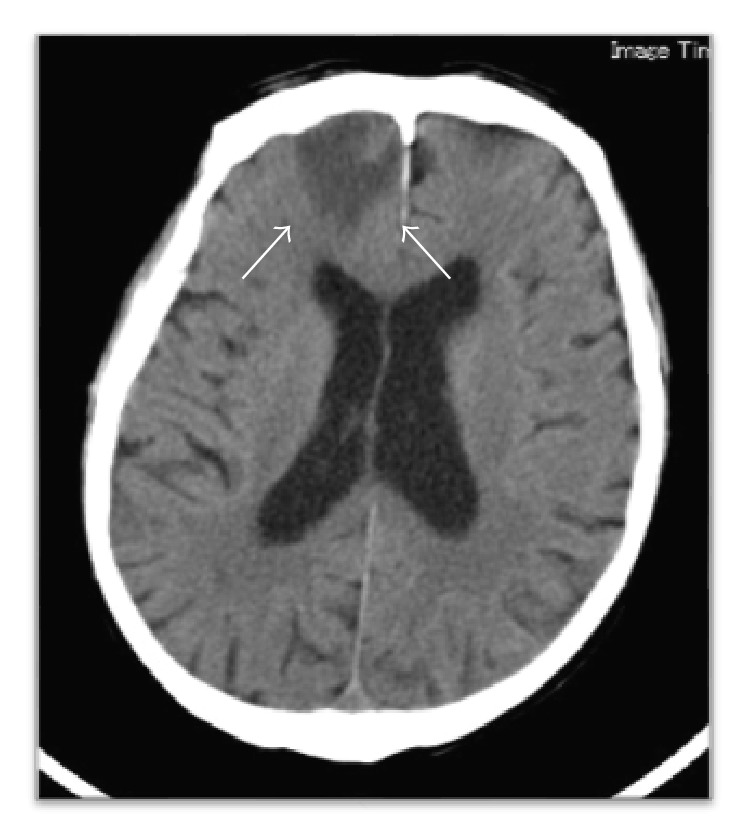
A CT showing a hypodense image in the right frontal lobe.
